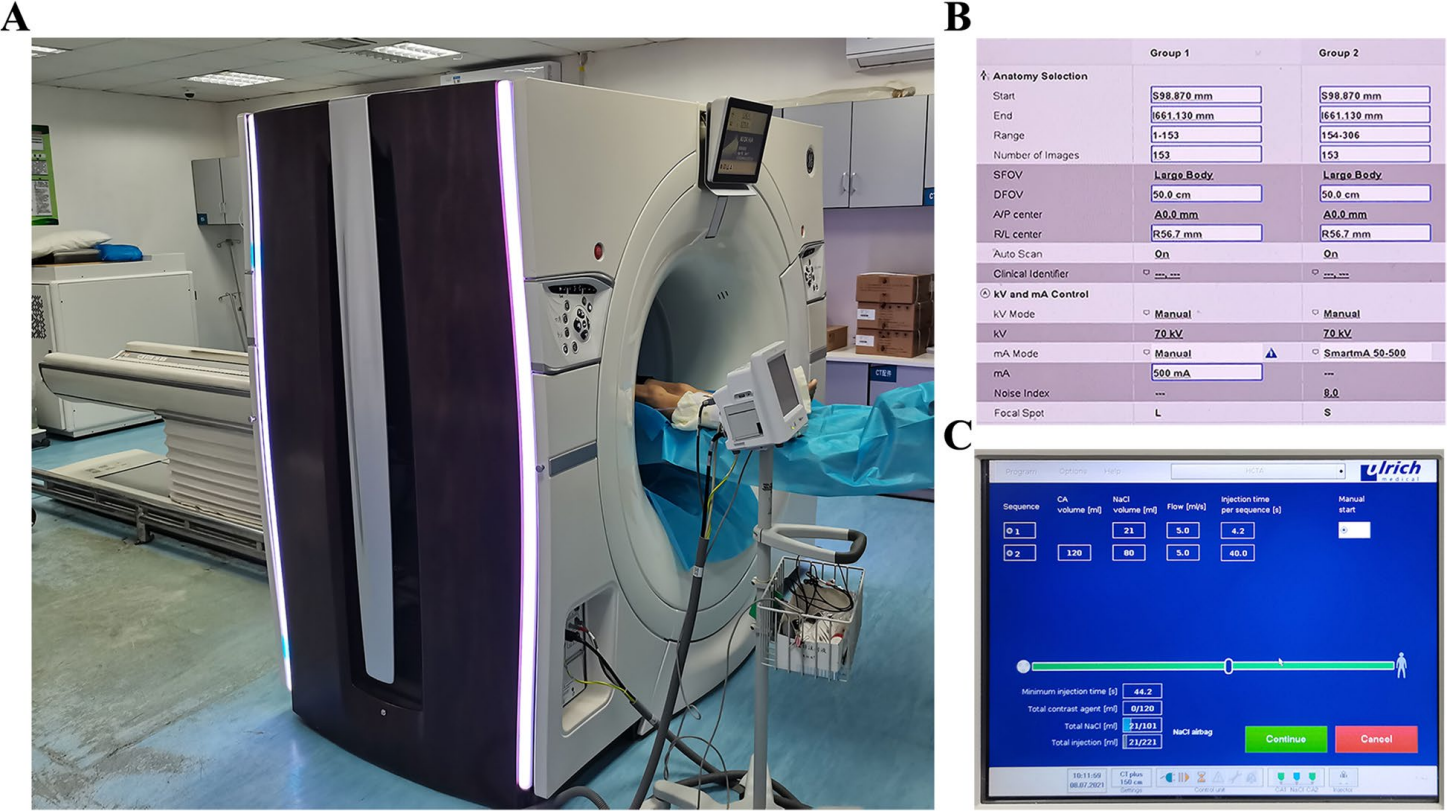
CTA examination and its parameters. A Patients were scanned by GE medical systems revolution CT. B The CTA parameters were set as lower kilovolt (70 kV) and higher mA value (500 mA). C The injection rate and total volume of iodinated contrast medium
